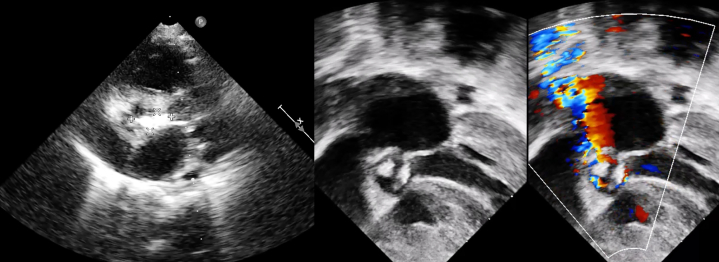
Preoperative Transthoracic Echocardiogram, Parasternal Long Axis View, Showing Aortic Valve Endocarditis•Severe aortic stenosis with peak/mean gradients of 88/42 mm Hg; absence of subaortic stenosis.•PAB in place with a peak gradient of 62 mm Hg, but preserved pulmonary trunk morphology without distorsion or torsion.•Competent pulmonary valve; no regurgitation.•No significant recoarctation.•Accelerated flow at the aortic isthmus with a peak Doppler gradient of 28 mm Hg.•Visualized tip of the VA shunt in the right atrium.
